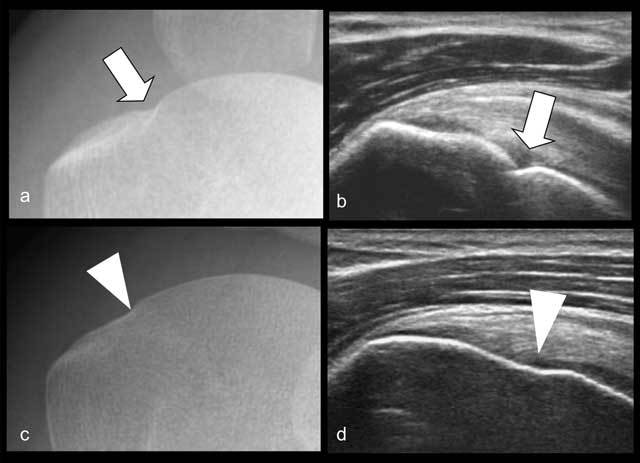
Interindividual variability of normal bony contours at the head-neck junction of the humeral head at radiography (a and c) and ultrasound (b and d). Marked depression (arrows in a, b) vs. smooth shallow depression (arrowheads in c, d).
